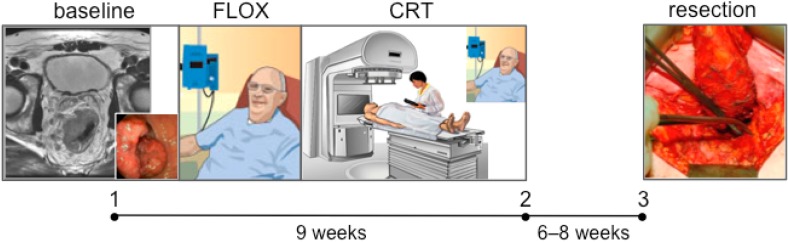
Locally Advanced Rectal Cancer—Radiation Response Prediction:* design of the circulating biomarker study. Serum samples (denoted by closed circles) were collected at baseline (*i.e.*, the time of diagnosis with rectal endoscopy and magnetic resonance imaging of the pelvic cavity; 1), following FLOX chemotherapy and chemoradiotherapy (CRT; 2), and before resection of the residual primary tumor (3). Treatment toxicity, assessed as detailed in Section 3, was recorded immediately following the completion of neoadjuvant treatment and repeatedly before the surgery. Images are from own work or adapted with permission [9,13].
